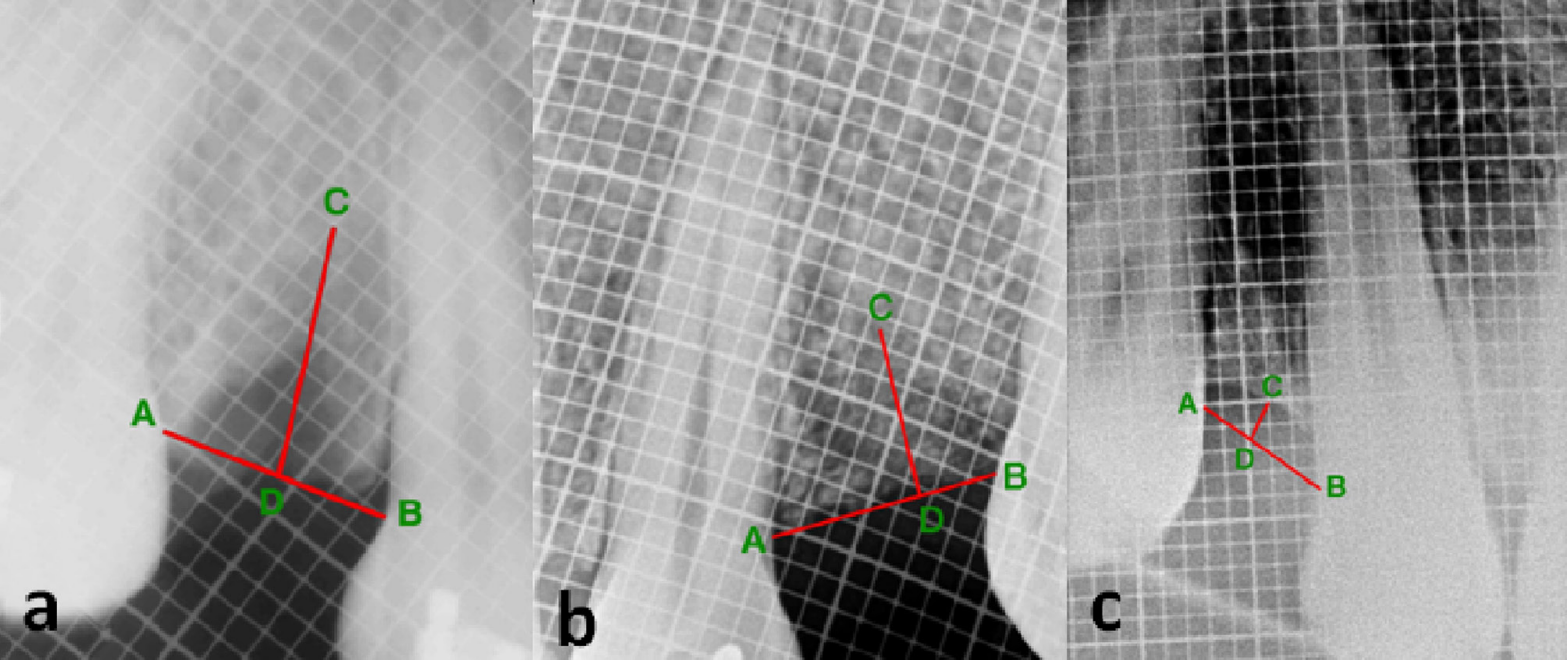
All the procedures were carried out under strict aseptic conditions. Following the administration of local anesthesia (2% LOX containing 2% lignocaine with 1:200000 adrenaline by Neon Laboratories Ltd.), the free gingival margin was elevated as conservatively as possible. The teeth were luxated using elevators and extracted atraumatically with forceps. After extraction, the sockets were carefully curetted. The patients’ arches (either maxillary or mandibular) were divided into quadrants and randomly labeled as test side and control side (in patients having even serial numbers, the right quadrant was labeled as the test side, and in patients with an odd serial number, the left quadrant was labeled as the test side). A collagen plug (KOLSPON PLUG) was inserted up to the crestal level in the extraction socket of the test side after saturating it with activated PRP and sutured into position using 3-0 silk sutures in the simple interrupted pattern. On the control side, the extraction socket was just sutured using 3-0 silk sutures in a simple interrupted pattern without placing any graft material (Figure 2). The width of the alveolar socket at the extraction site was measured using bone calipers at three levels (crestal, mid-root, and apical), immediately after extraction and after 3 and 6 months. A digital radiograph (RVG) was taken in combination with a radiopaque millimeter-graduated grid to evaluate the baseline bone height immediately after extraction and after 3 and 6 months. The extraction of the tooth and radiographic assessment were carried out by two different operators. The patients were given routine antibiotics and analgesics (Amoxicillin tablets, 500 mg TID, and Diclofenac sodium tablets, 50 mg BD for three consecutive days) and recalled 10 days after the surgery for suture removal and evaluation of soft tissue healing according to Landry, Turnbull, and Howley index.^20^
